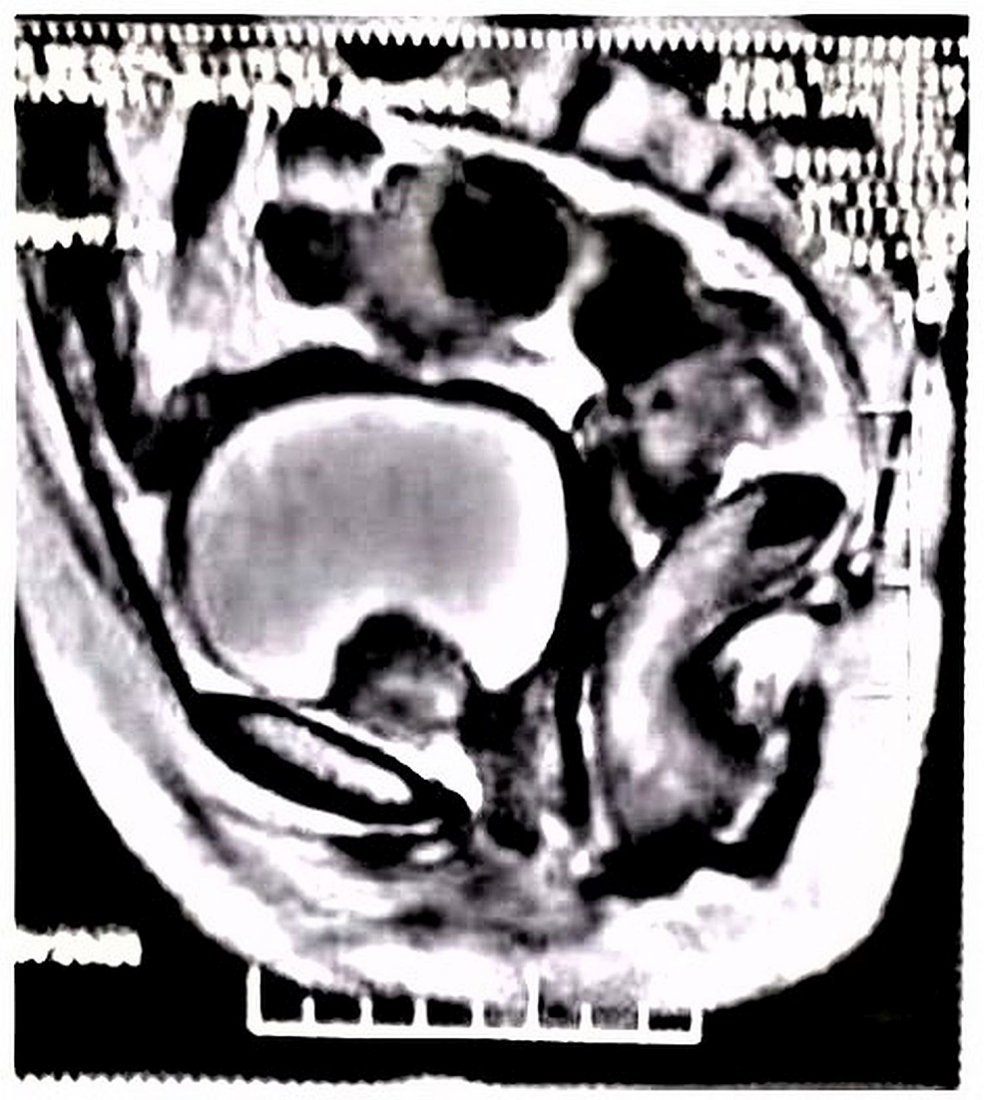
Contrast-enhanced computed tomography (CECT) image - Lobulated tumor mass arising from the base of urinary bladder and protruding into the cavity.
